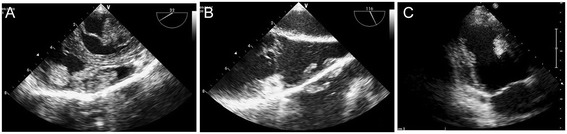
Identification of cardiac myxomas by echocardiogram. (A) Patient 1 - pre-operative myxomas in the left atrium and right ventricle. (B) Patient 1 - pre-operative myxomas in the left ventricle. (C) Patient 2 - post-operative recurrent myxoma in the left ventricle 3 months after the operation.
